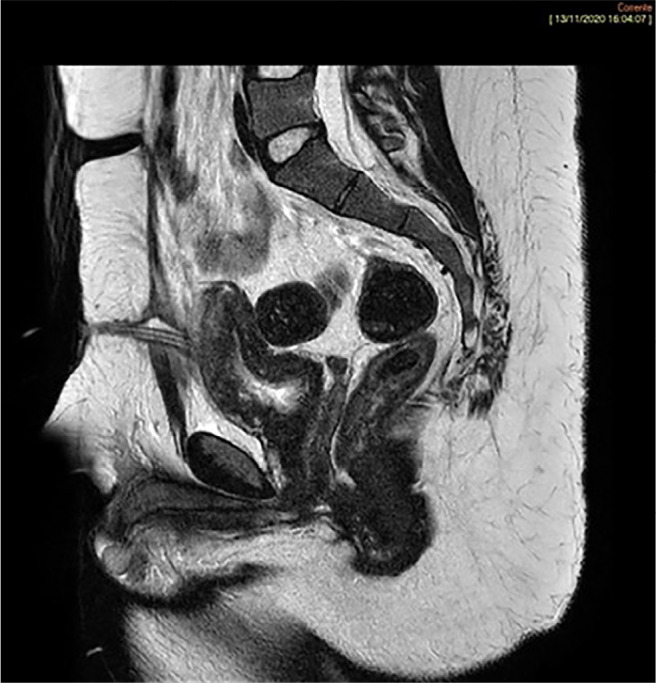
T2-weighted sagittal image: Uterine remnants between rectum and bladder
